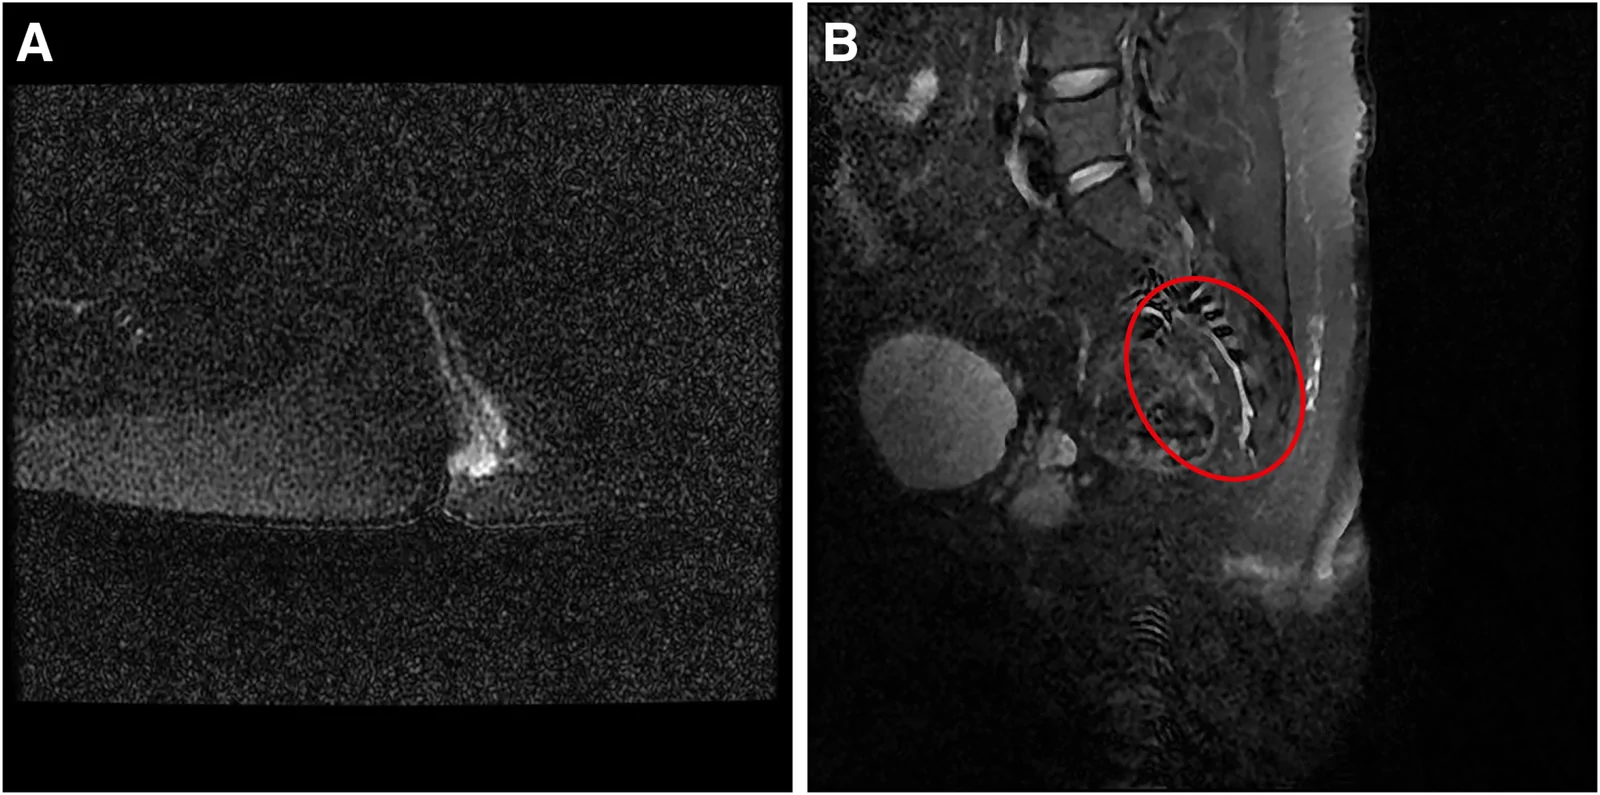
Initial pelvic MRI showing suspected susceptibility-related artifacts. (A) Oblique axial diffusion-weighted image showing diffuse signal loss in the sacrococcygeal region. (B) Oblique sagittal T2-weighted STIR image showing radial signal-loss artifacts (red oval). MRI = magnetic resonance imaging, STIR = short tau inversion recovery.
